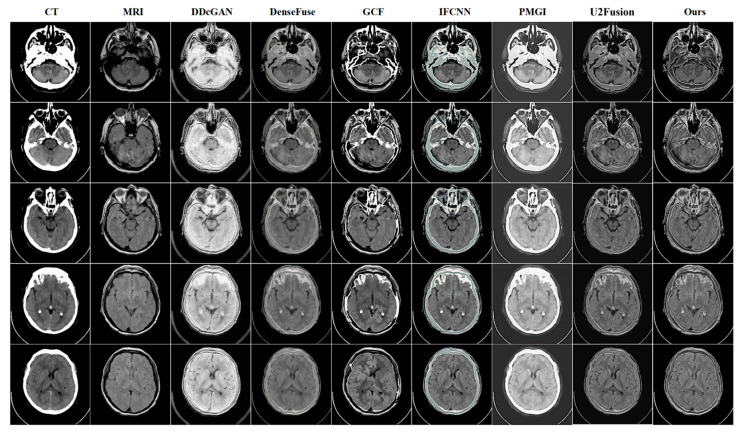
Fusion result of CT-MRI.
